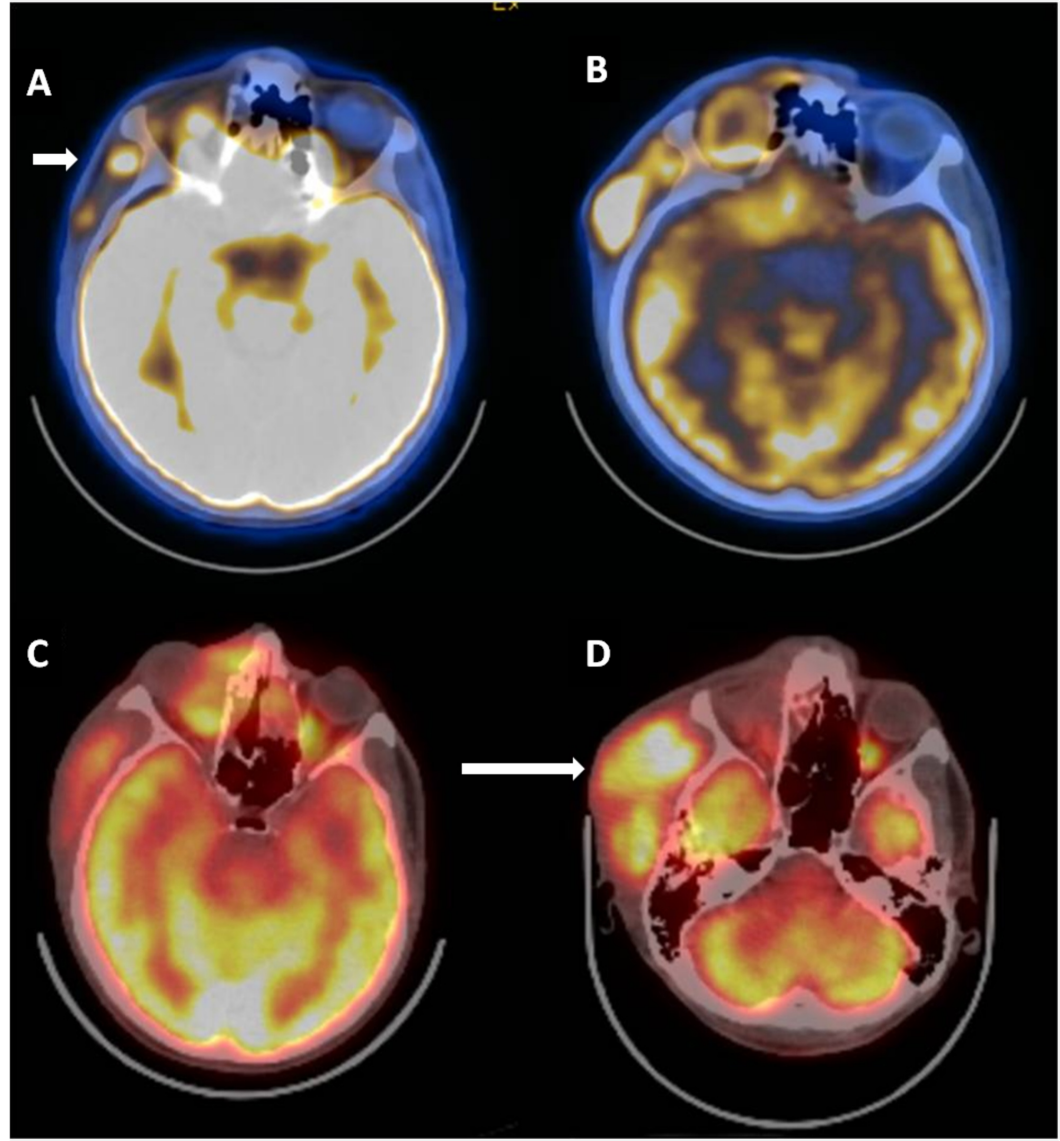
Positron emission computed tomography (PET-CT) performed before, during, and after chemotherapy for recurrence showing increased fluorodeoxyglucose (DG) uptake 1A. PET-CT performed three months after primary radiotherapy showing an FDG avid recurrent lesion (short arrow) in the right pterion and right orbit and in the lateral side of the zygomatic bone. 1B. PET-CT performed after two cycles of docetaxel/ cisplatin and six cycles of gemcitabine in combination with oxaliplatin showing the progressive lesion. 1C. PET-CT performed after four cycles of pembrolizumab showing a partial response in the primary site. 1D. PET-CT performed after eight cycles of pembrolizumab showing progressive disease (long arrow).
